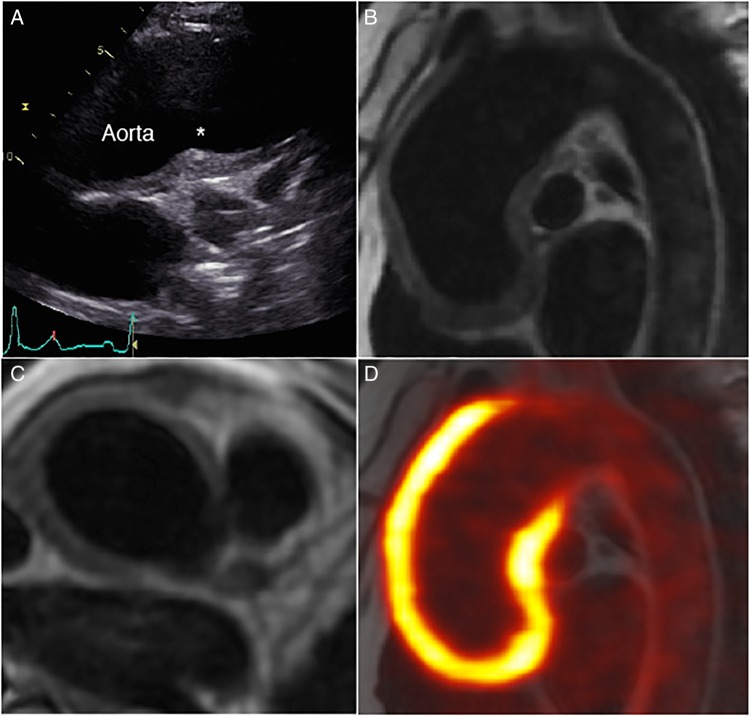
Transthoracic echocardiogram in high left parasternal long-axis view (A); cardiac MRI with black-blood sequence in sagittal (B) and axial planes (C); fused ^18^F-fluorodeoxyglucose positron emission tomography-MRI (D). The asterisk in 1A refers to para-aortic thickening.
